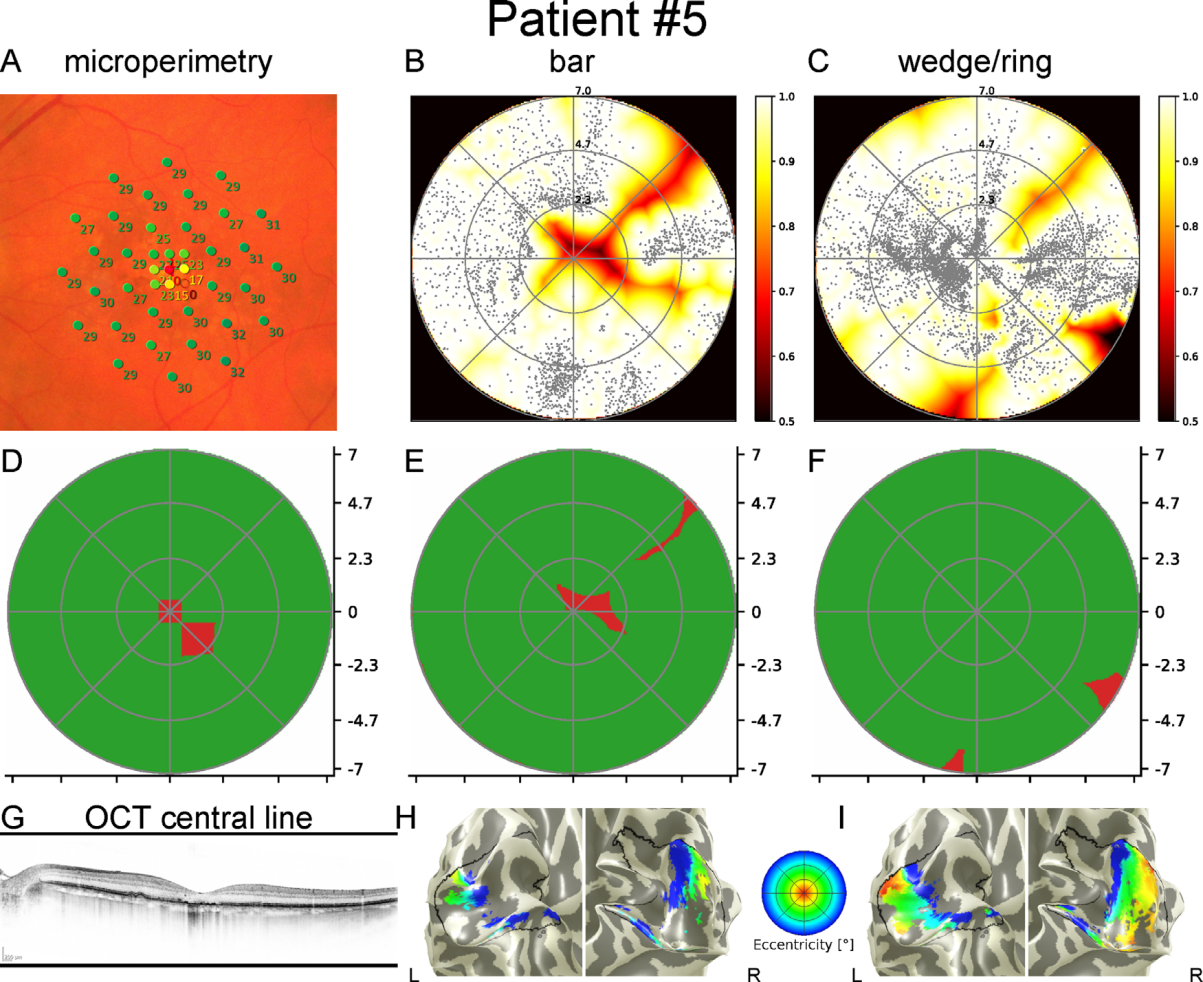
Results of the left eye of patient 05 with a small parafoveal scotoma secondary to geographic atrophy. *Top row*: Superposition of MP on the fundus image where macular sensitivity loss is visible (A) (point colors *red* to *green* indicate 0 dB to 34 dB MP results); pRF coverage maps calculated from the functional MRI data with bar (B) and wedge/ring stimuli (C); *Middle row*: binarized MP (D); binarized pRF coverage maps from bar (E) and wedge/ring stimuli (F). *Bottom row*: Central OCT (G); eccentricity maps from bar (H) and wedge/ring stimuli (I) overlaid on the white matter/grey matter surface mesh. There is a strong agreement between the pRF map based on bar stimuli and MP. However, in the maps based on wedge/ring stimuli, the scotoma is concealed by the central clustering of pRF centers (bar: <2.5 degrees: 196 [9%], >2.5 degrees: 2090 [91%]; wedge/ring: <2.5 degrees 2358 [44%], and >2.5 degrees: 3022 [56%]) and at worst fails to reveal a scotoma. SMC values for this patient are 0.80 (<2.5 degrees) and 0.99 (>2.5 degrees) for bar stimuli and for wedge/ring stimuli 0.85 (<2.5 degrees) and 0.99 (>2.5 degrees), respectively. Due to a small shift in position of the scotoma, SMC values are smaller for the center of the visual field in maps made using bar stimuli even though it is entirely missing in the wedge/ring analogue.
